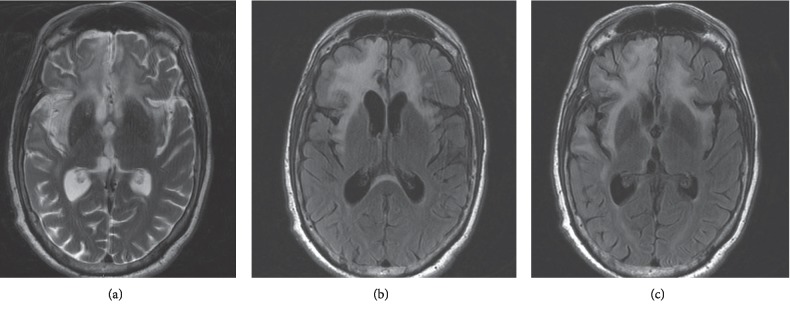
Brain MRI obtained during admission for anti-NMDAR encephalitis, 8 months after HSE onset. Increased T2 (a) and T2/FLAIR (b, c) signal (hyperintensity) in bilatéral frontaltemporal lobes (predominant on the right side).
